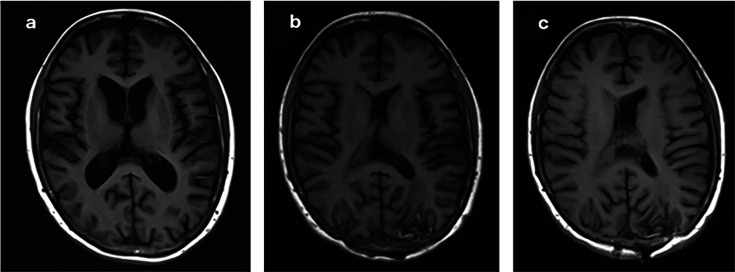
Serial MRIs of the patient’s midline mass centered between the bodies of the lateral ventricles with findings most concerning for a neoplastic process: (a) MRI from September 2023; (b) MRI from September 2024; (c) MRI from January 2025.
